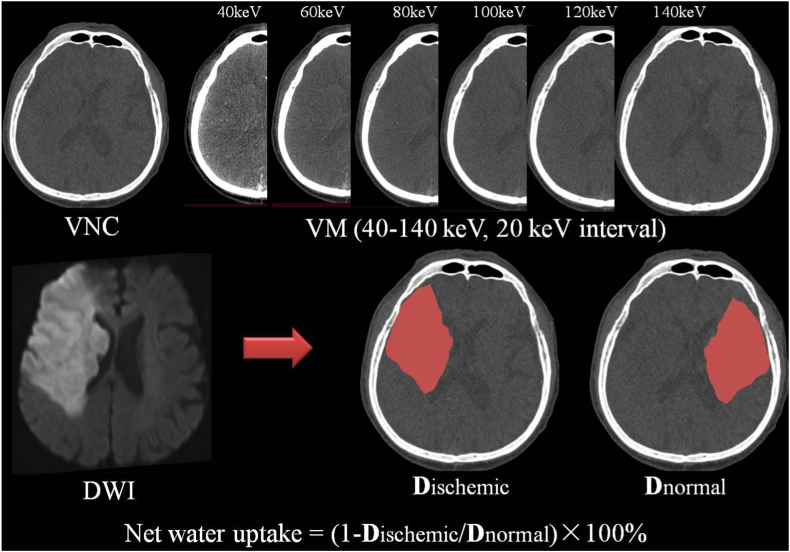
Determination of % net water uptake (NWU) per volume of early infarct on virtual images derived from DECT angiography. The early infarct (i.e., the core lesion) was identified with the reference of DWI. The mean CT density of the infarct core (D*ischemic*) was then calculated in relation to the normal CT density (D*normal*) measured on a mirrored contralateral healthy brain region of interest.
